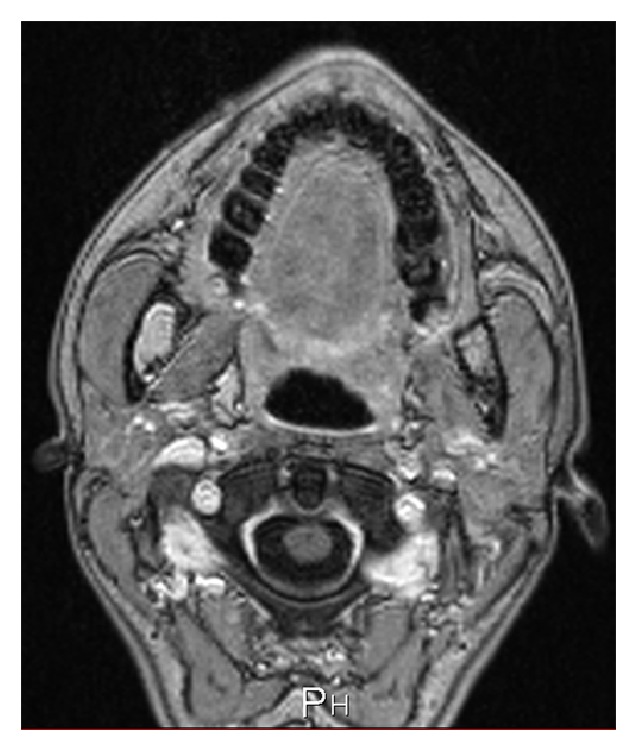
MRI showed the lesion with signal reduction in the sequences with fat suppression.
